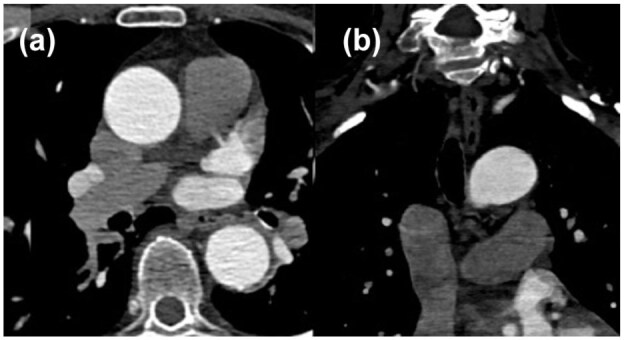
Indeterminate CTA imaging for LAA thrombus on axial (a) and coronal (b) sequences.
